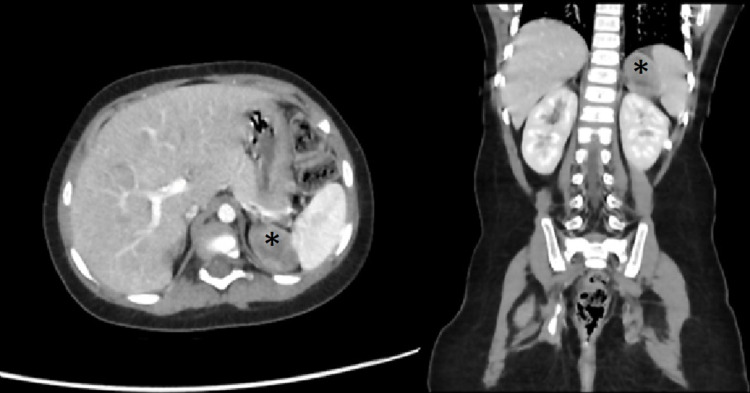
CT of the chest, abdomen, and pelvis detected a left suprarenal oval-shaped mass measuring 3.6 × 3.3 × 2.3 cm. CT: computed tomography
